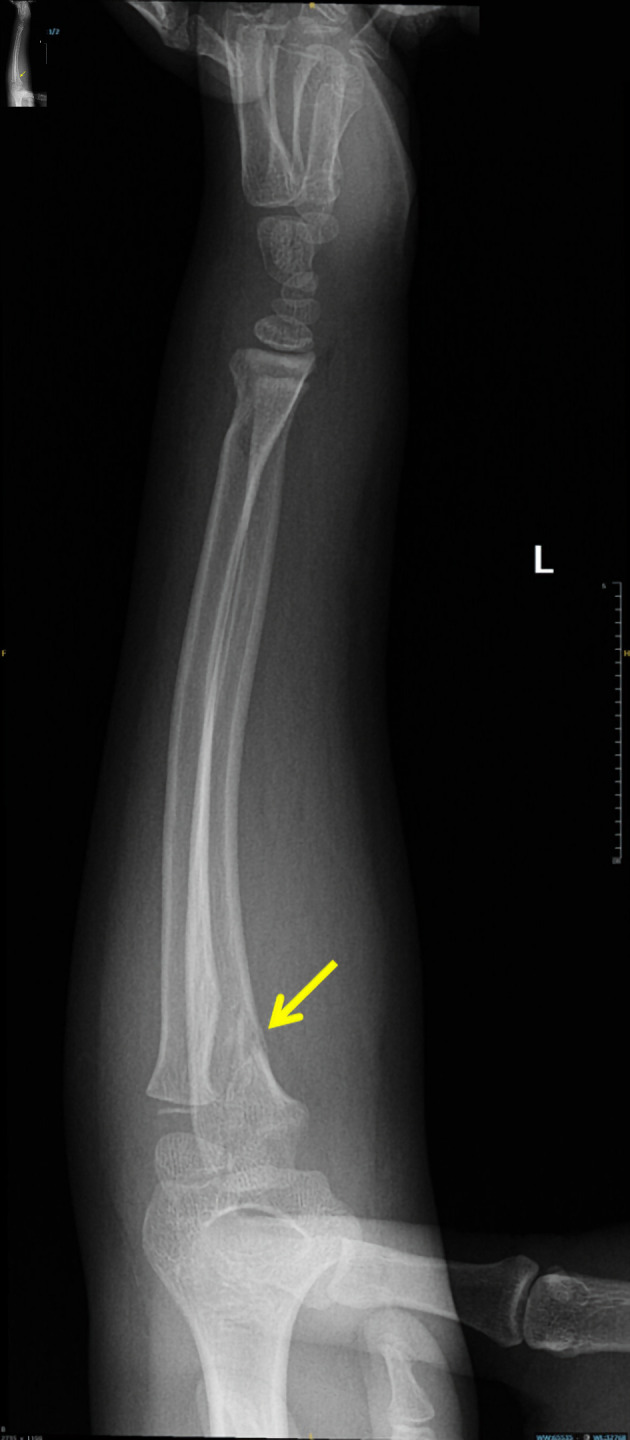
Anteroposterior forearm radiograph demonstrating proximal ulnar fracture (arrow).
